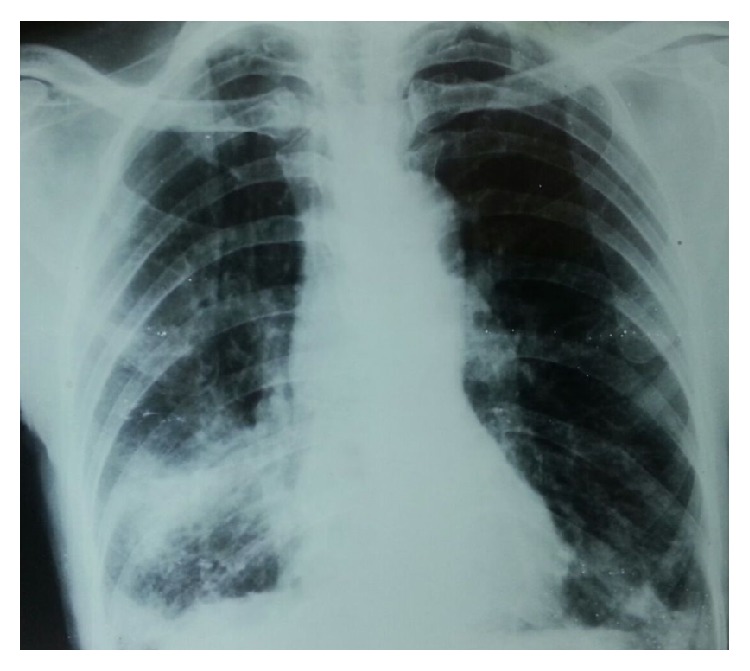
Chest X-ray with minimally advanced lesion.
